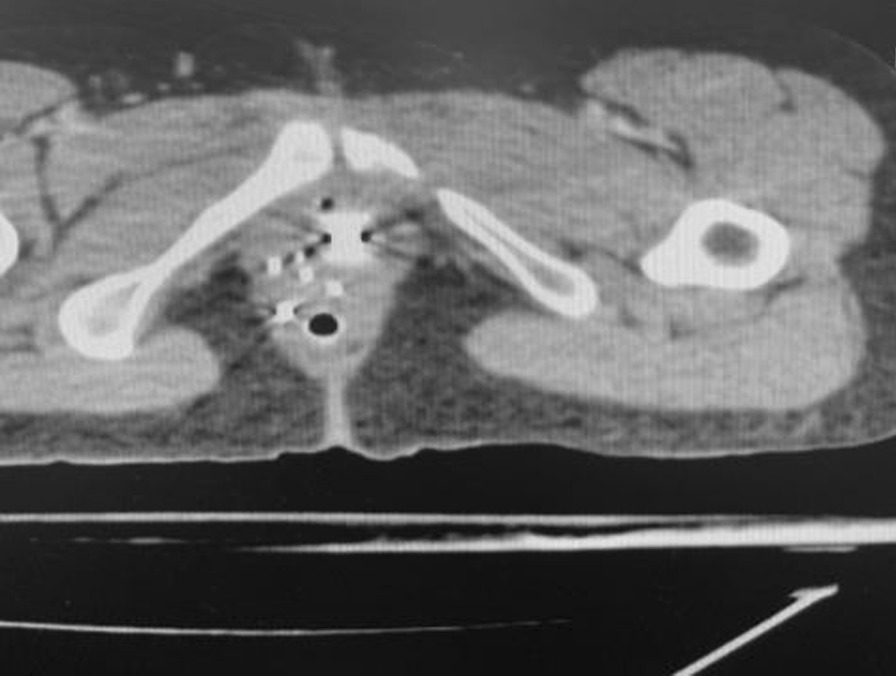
The axial view of the patient’s computed tomography scan after interstitial catheter insertion
